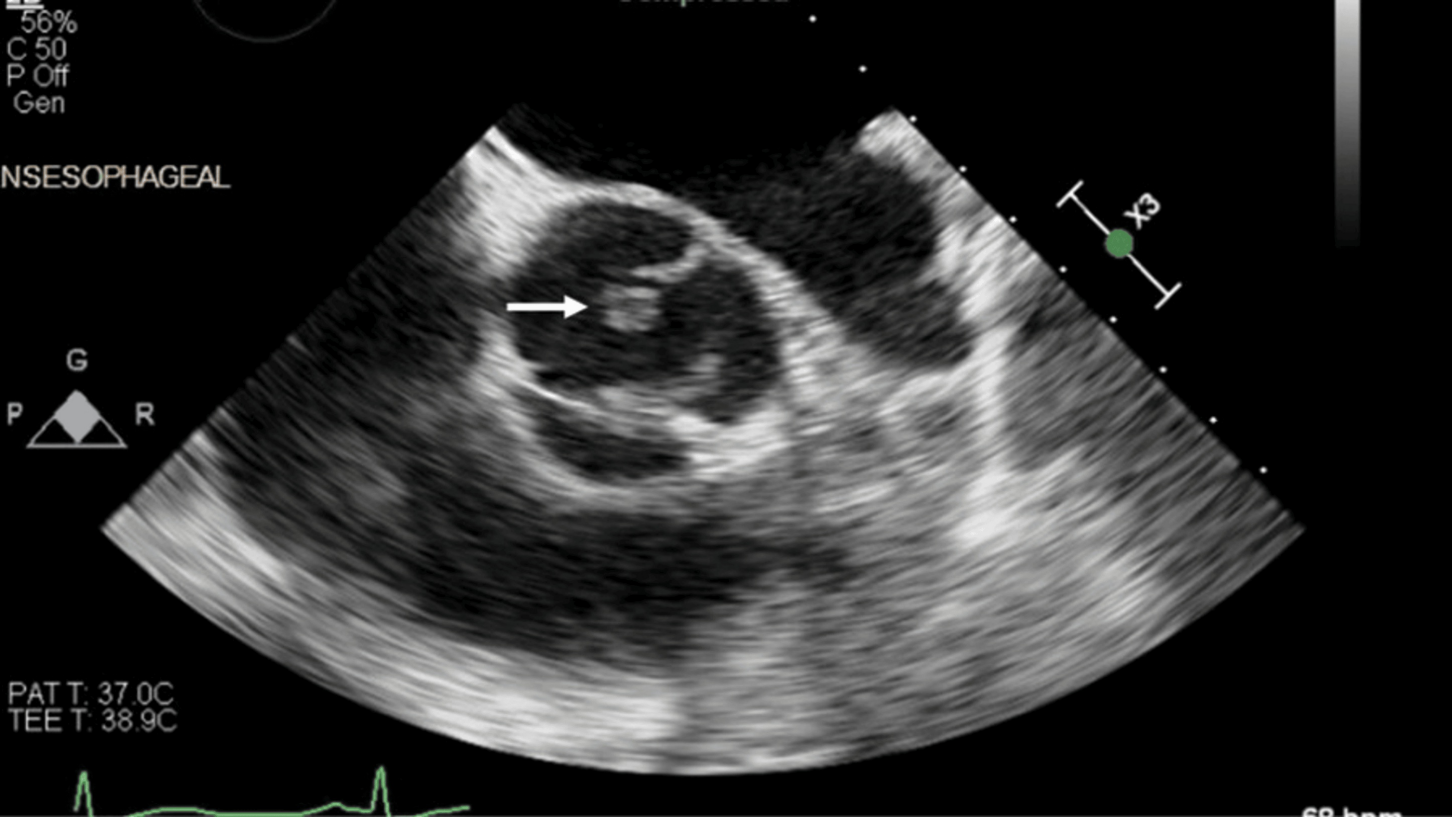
TEE in the mid-esophageal aortic valve short-axis view TEE, transesophageal echocardiogram
